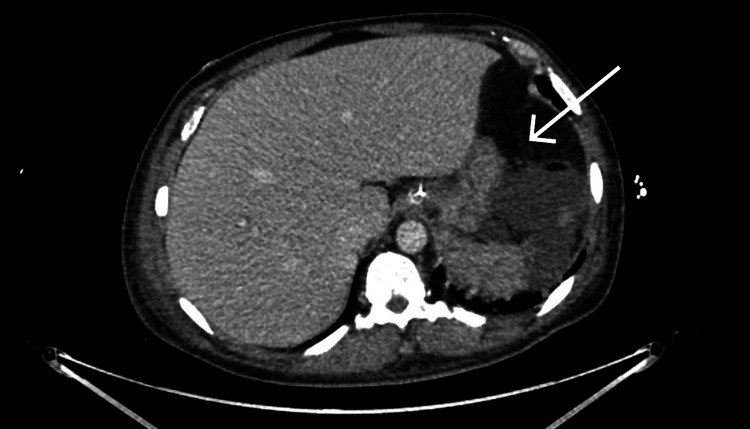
Computed tomography (CT) abdomen with contrast, arrow showing splenic abscess
